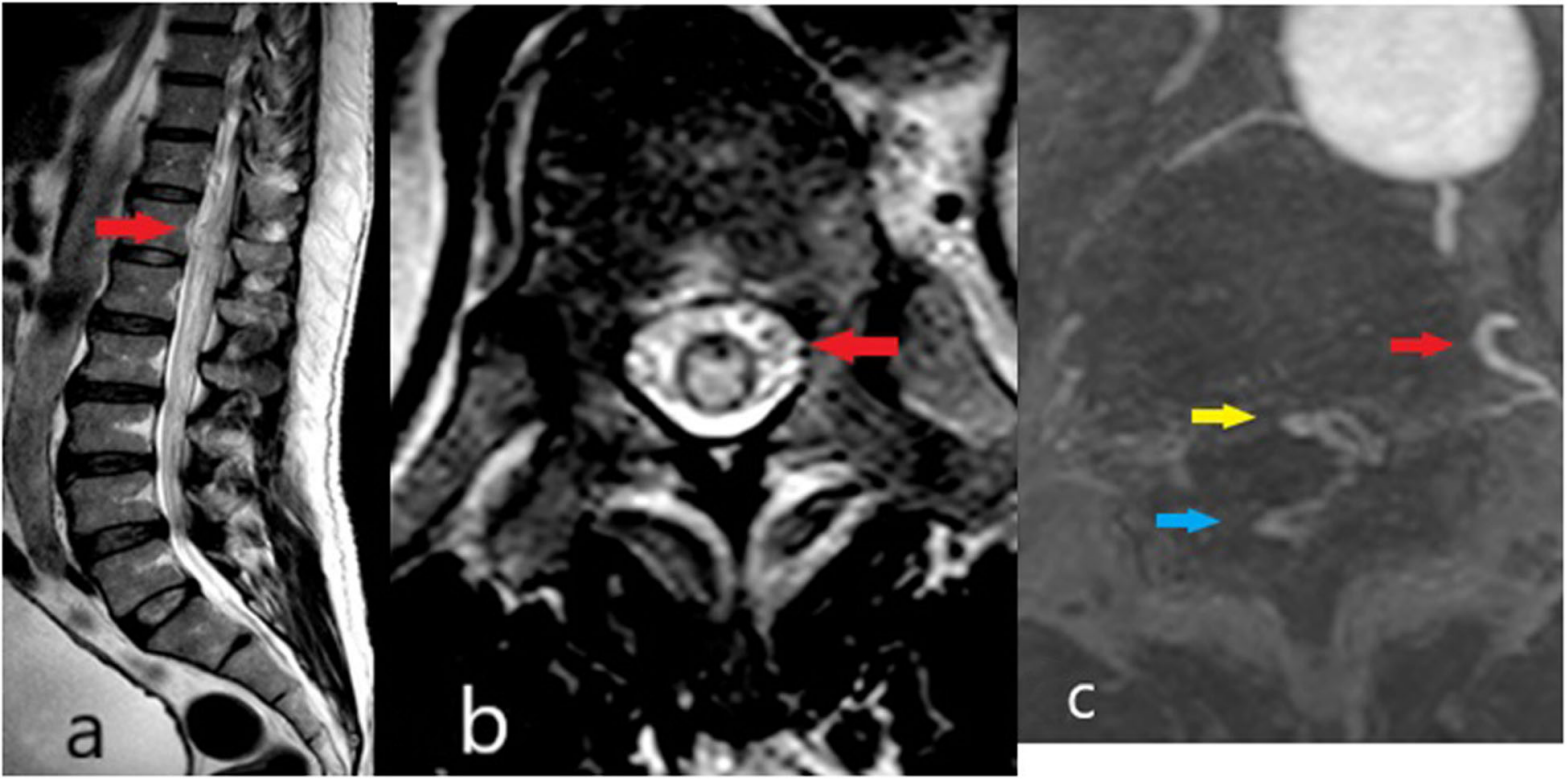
a and b MRI spine of patient 4 showing the suspicious dilated vessels at the thoracolumbar cord (red arrow). c. CT angiogram (row below) demonstrated the feeding vessel (red arrow), dural AVF (yellow arrow) and the dilated perimedullary vein (blue arrow)
